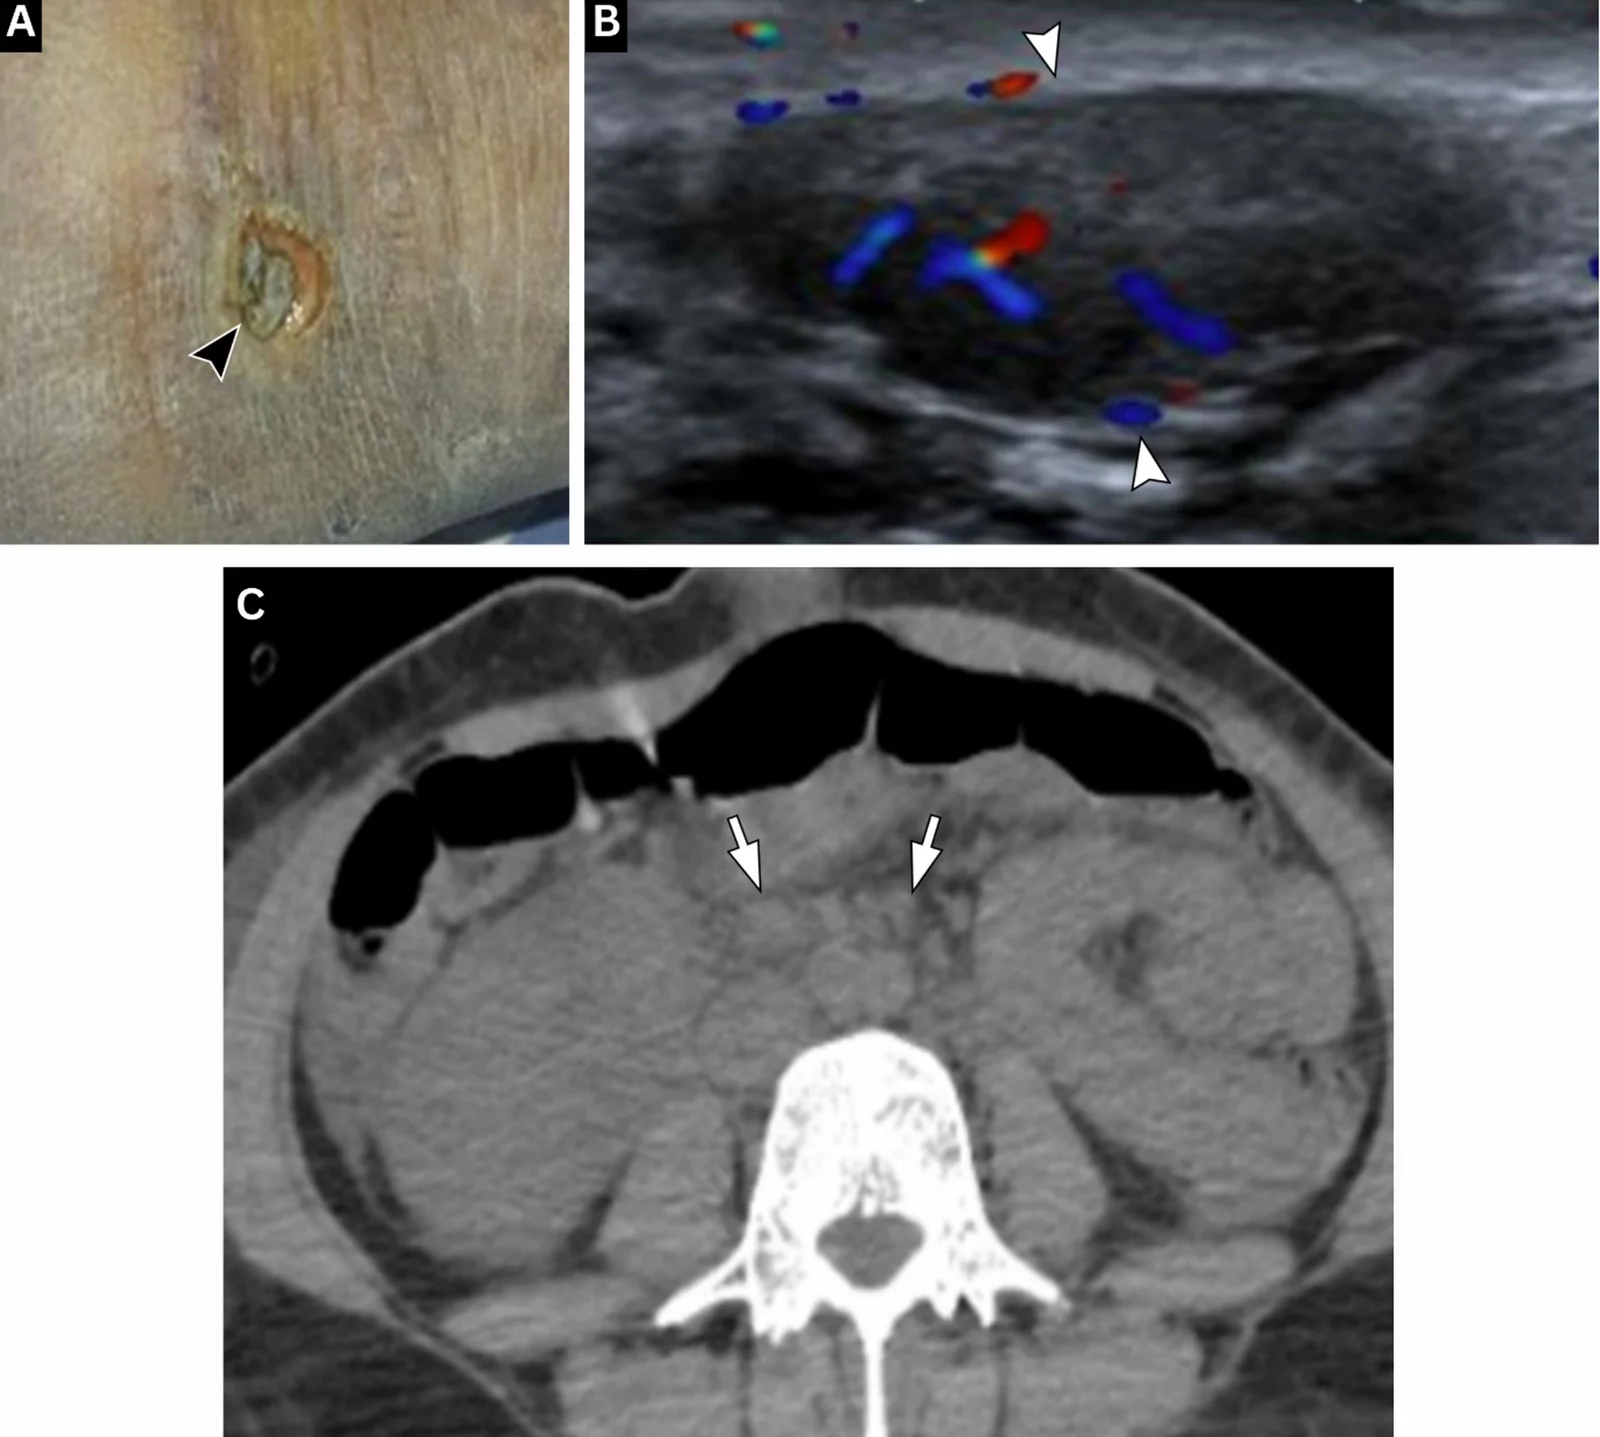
A, B Clinical photograph shows an eschar over the left heel (black arrowhead, A) in a 45-year-old male who presented with fever and myalgia since 4 days. B Ultrasound demonstrates an enlarged left inguinal lymph node (white arrowheads) with preserved fatty hilum (regional lymphadenopathy). C Noncontrast CT shows mesenteric lymphadenopathy (solid white arrows) in a 29-year-old male who presented with pneumonia and acute kidney injury, which was subsequently attributed to scrub typhus
